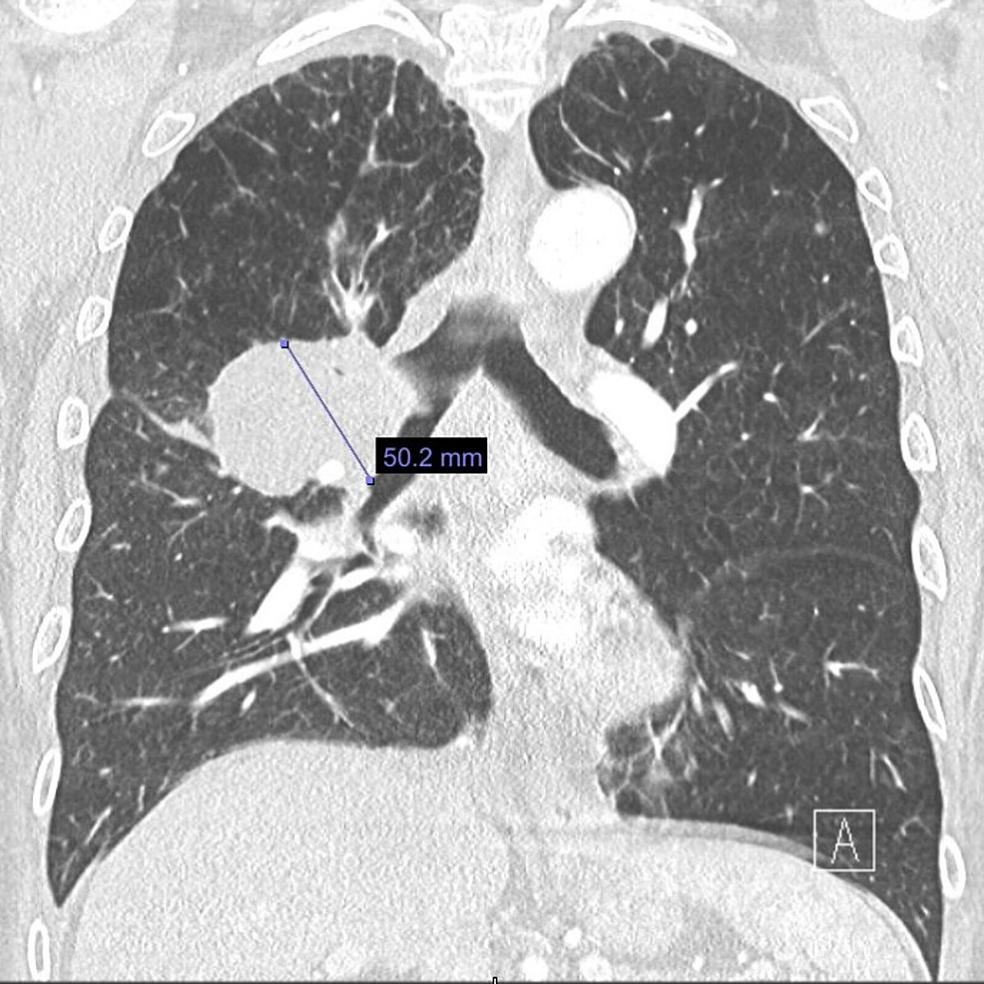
CT scan of the chest post-chemotherapy showing regression of the lung tumor burden. CT: computed tomography
